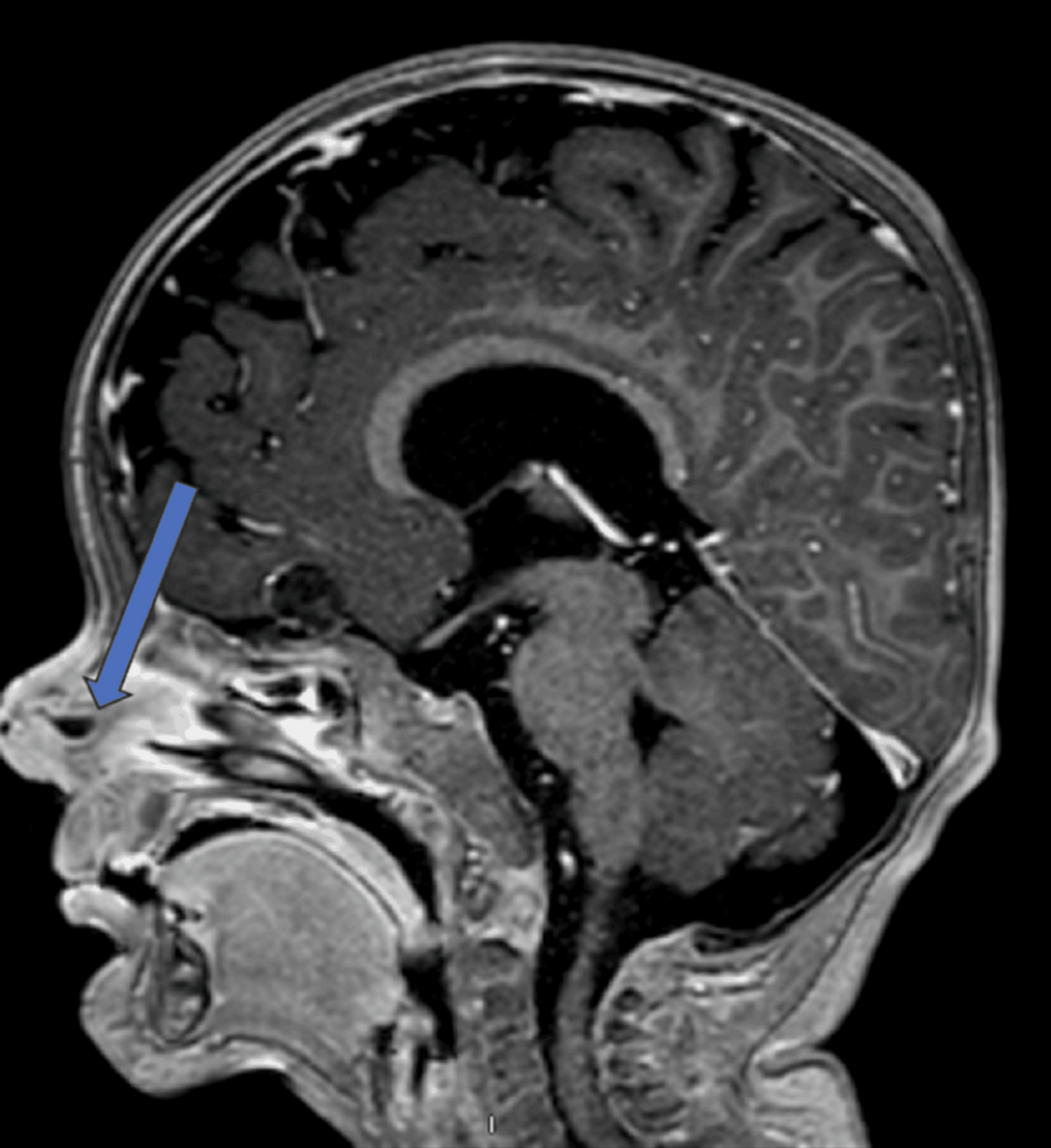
T1 sagittal post contrast MRI demonstrating blind ending of lesion. MRI, magnetic resonance imaging
